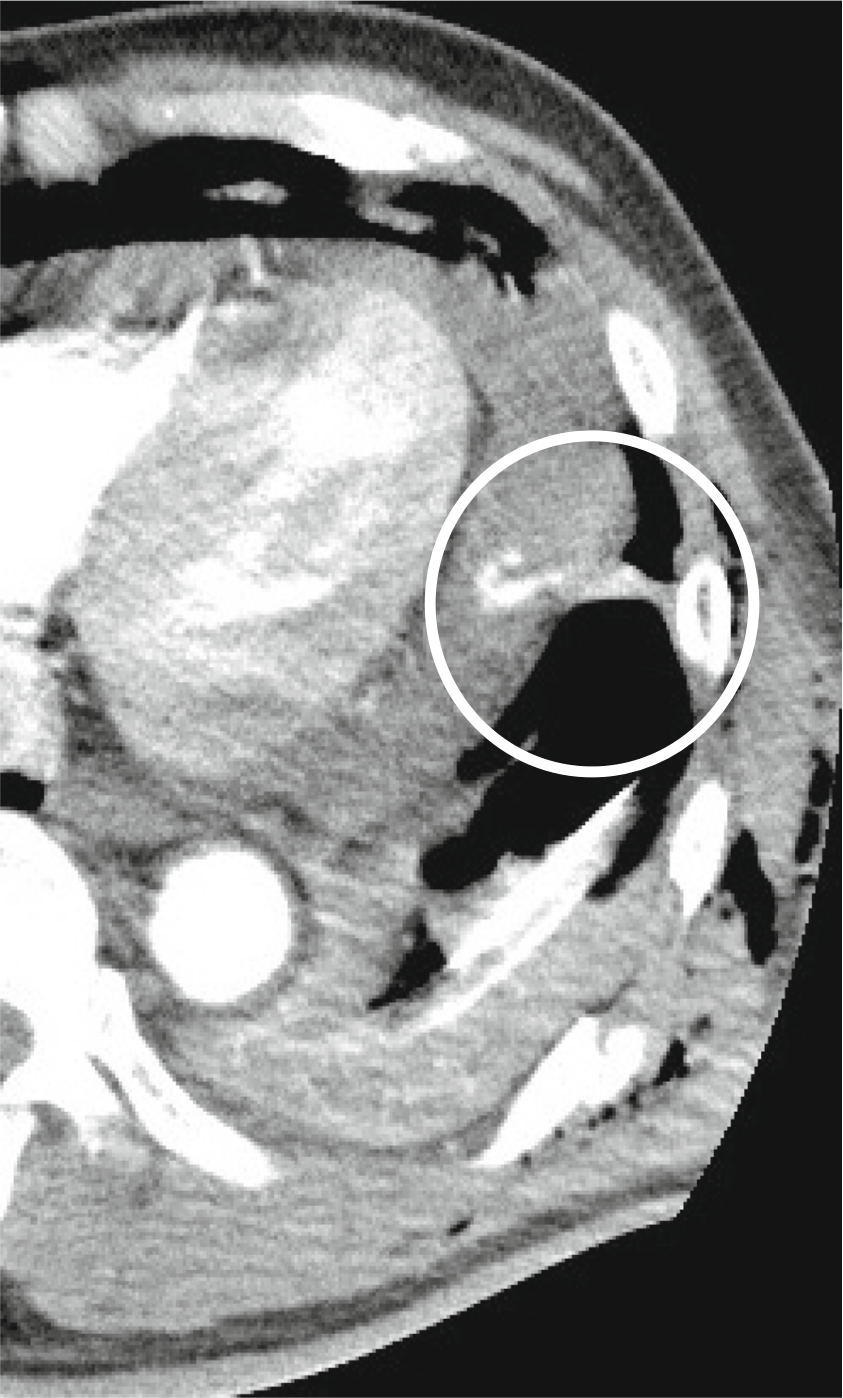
Dynamic CT scan finding at 10 h after admission. Dynamic CT scan showed active bleeding in the pericardium (circles)
